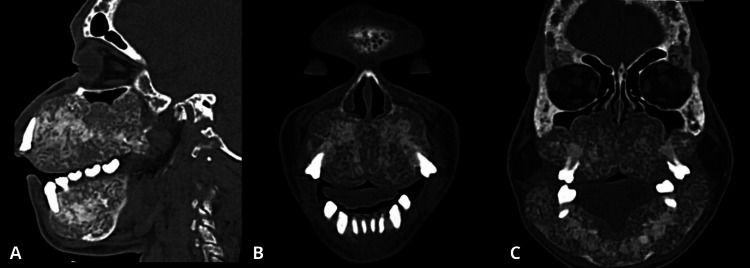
Head CT A: Sagittal view of maxilla displaying overgrowth of maxillary and mandibular bones with extensive lesions with a lithic appearance. B: Coronal view of the face at the nasal septum level showing diffuse enlargement of the hard palate with superimposed bony permeative change and spaced teeth. C: Coronal section at the level of the orbital cavity showing overgrowth of the maxilla and mandible bones profoundly affected by diffuse bone abnormalities CT: computed tomography
